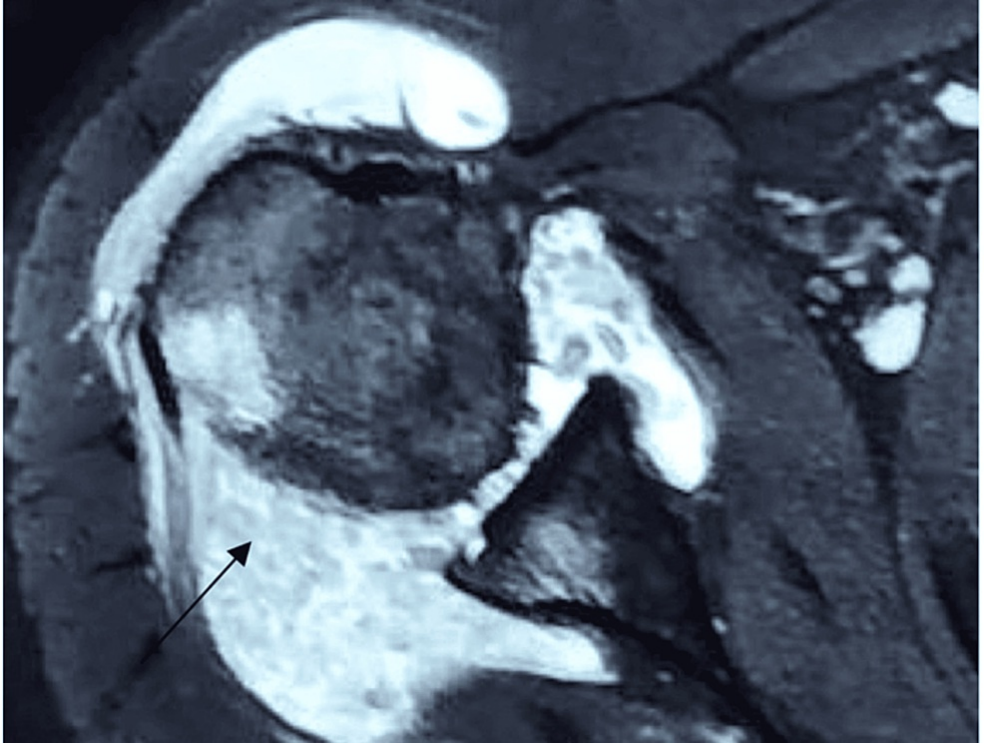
MRI revealing smooth erosion into the posterolateral aspect of the humeral head, indicative of pigmented villonodular synovitis
